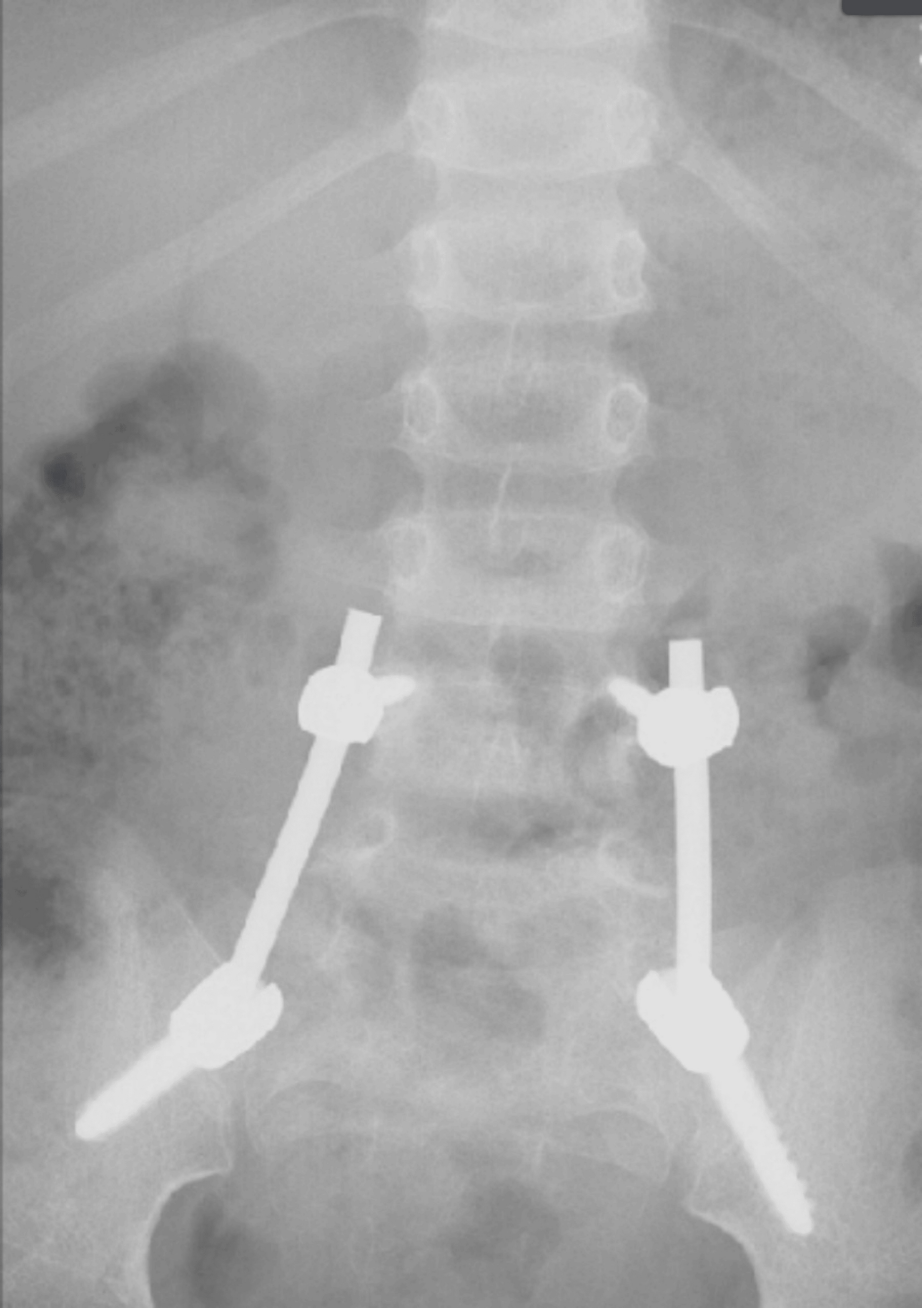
Radiograph at three months postoperatively showing good clinical progression
